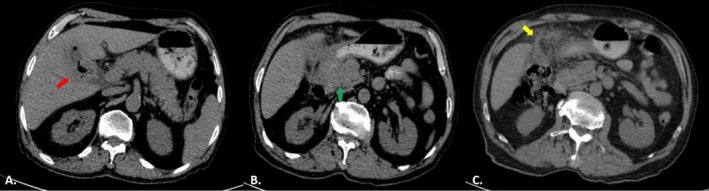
Abdominopelvic CT scan without IV contrast. (A) Gallbladder has an indistinct margin and contains intraluminal air foci (red arrow), suggestive of a cholecystoduodenal fistula. (B) Hypodense ill‐defined mass in pancreatic head with invasion into the adjacent duodenum (green arrow). (C) Fat stranding and soft tissue density in mesentery of hepatic flexure of colon (yellow arrow), suggestive of mesenteric invasion.
